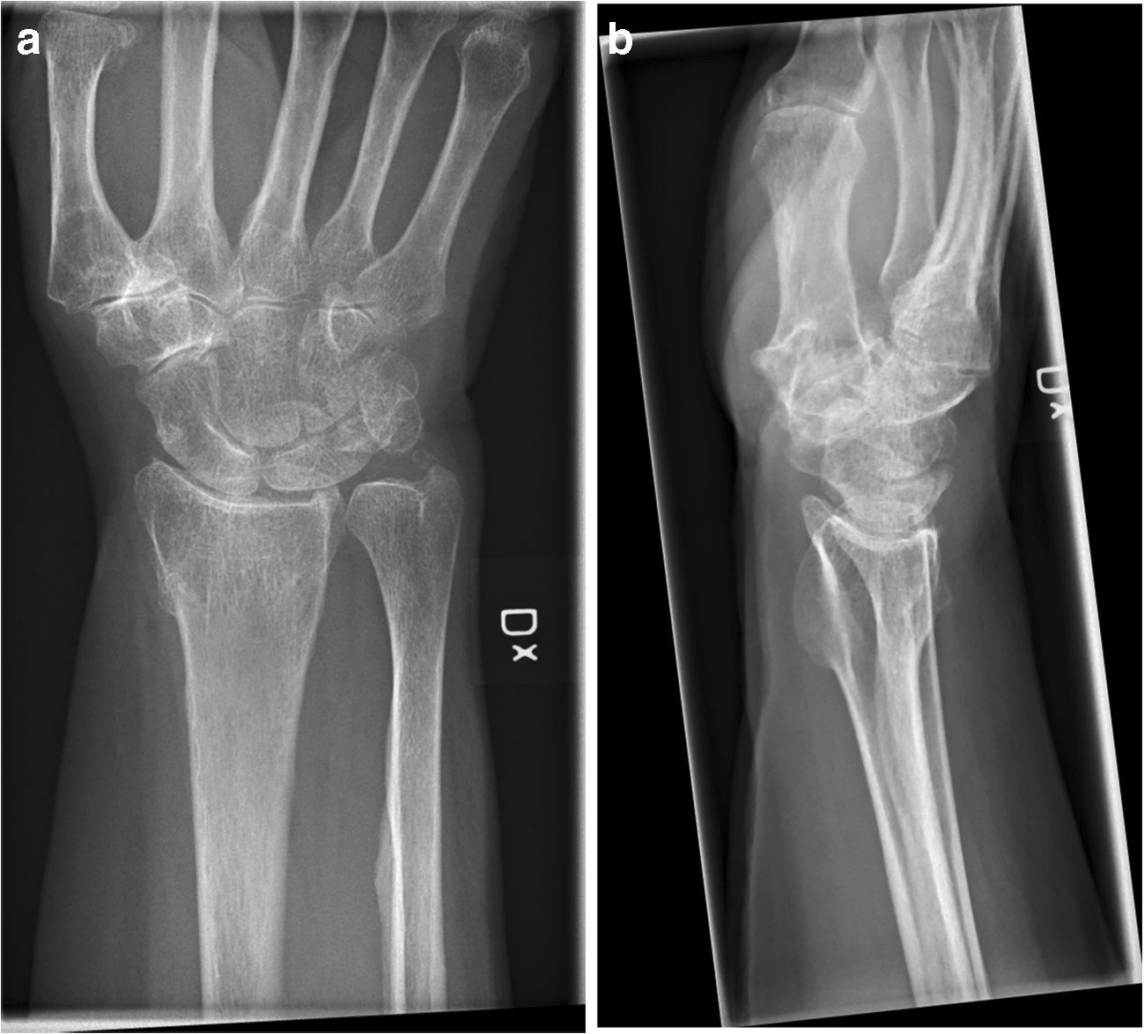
Pre-operative radiograph of malunited distal radial fracture. a AP view. b Sagittal view
